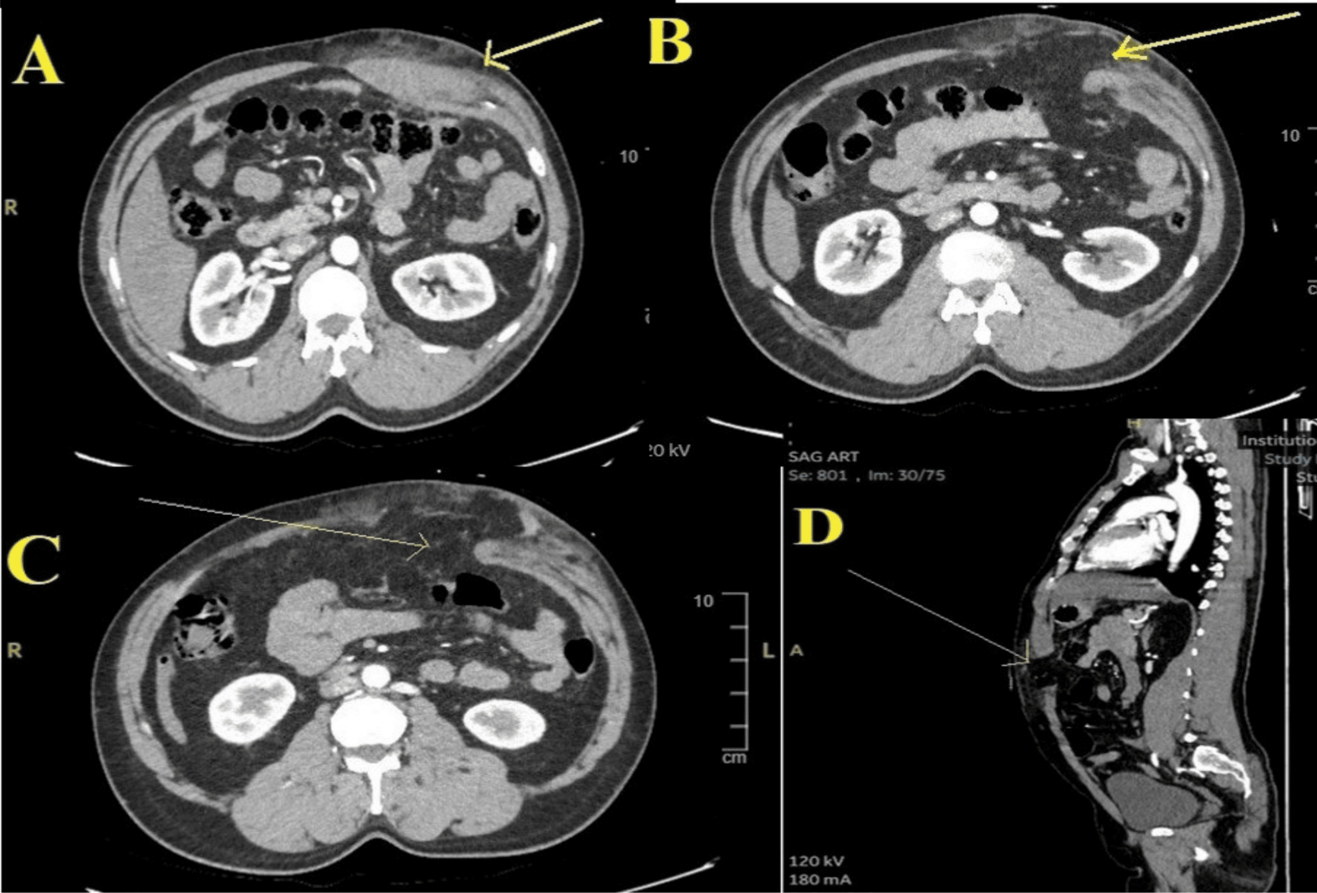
CT scans revealing abdominal wall hematoma and defect. The images show (A) hematoma in the abdominal wall muscle, axial view (arrow); (B and C) abdominal wall defect with intact skin, axial view (arrow); and (D) abdominal wall defect, sagittal view (arrow).
